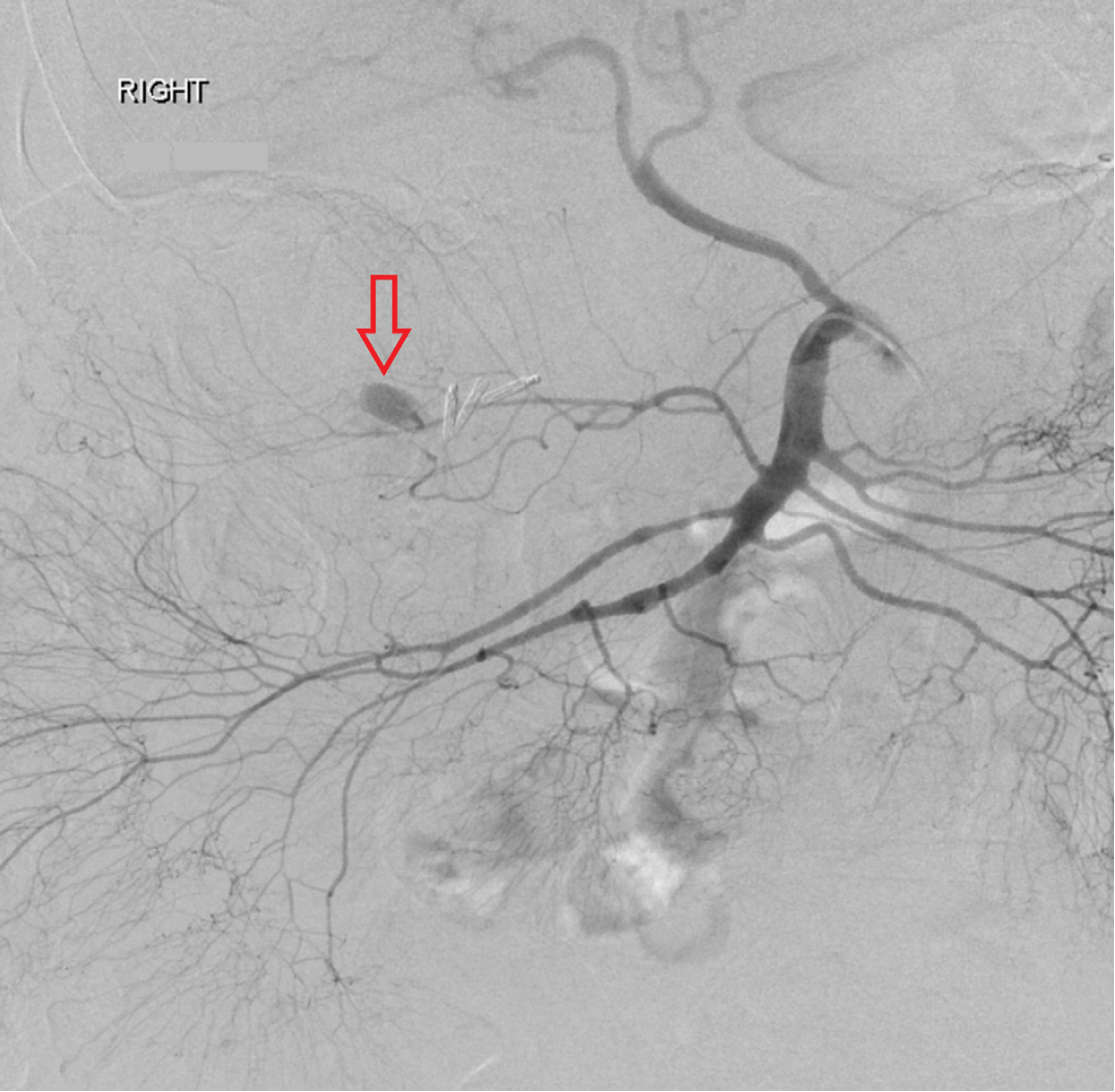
Following a 40‐min delay experienced between computed tomography (CT) and digital subtraction angiogram (DSA) imaging, the same bleed is observed on PA DSA imaging. Previous cholecystectomy clips are noted in the region of the bleed.
